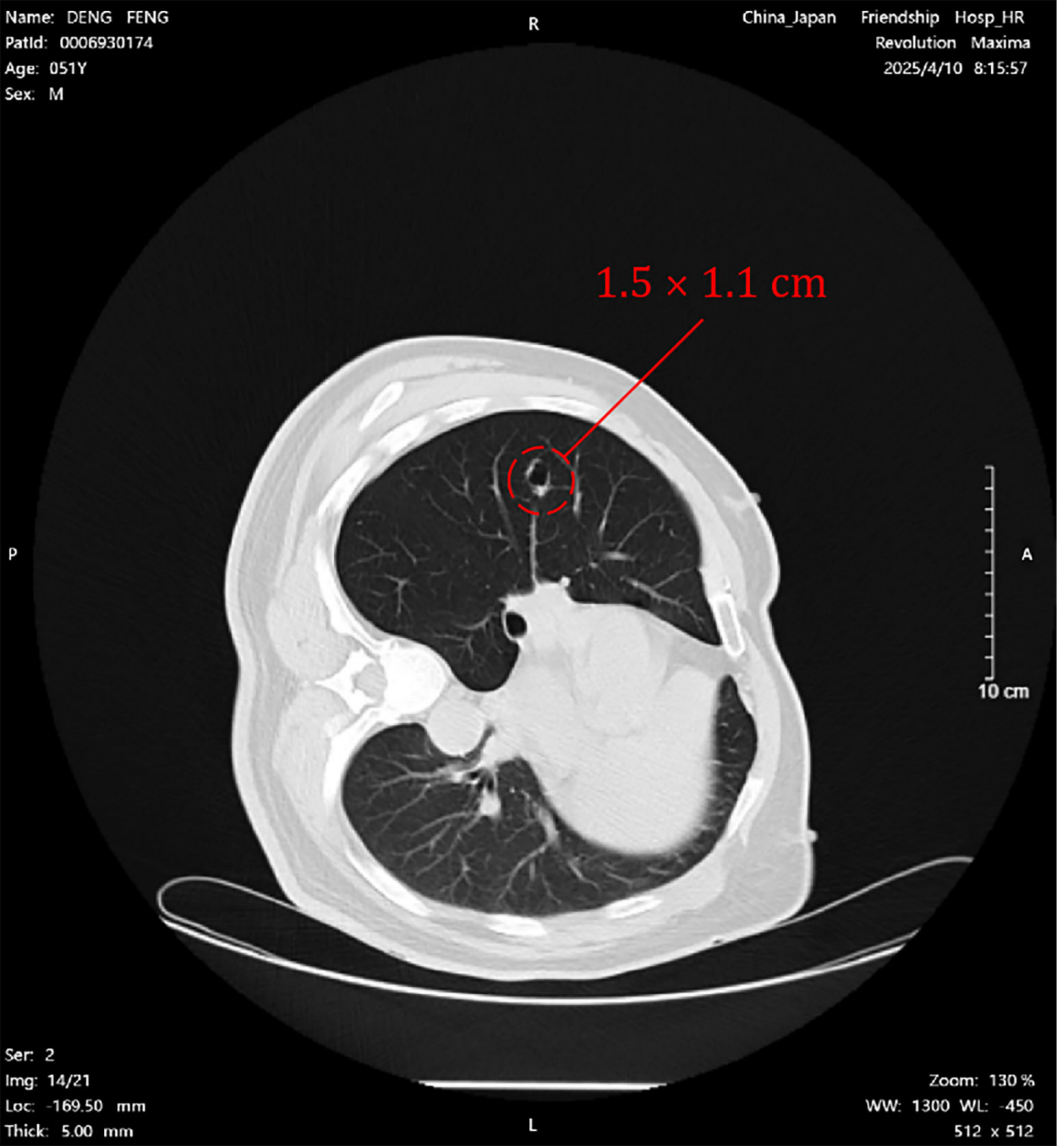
Chest PET-CT revealed a cystic cavity, measuring approximately 1.5 cm × 1.1 cm.
